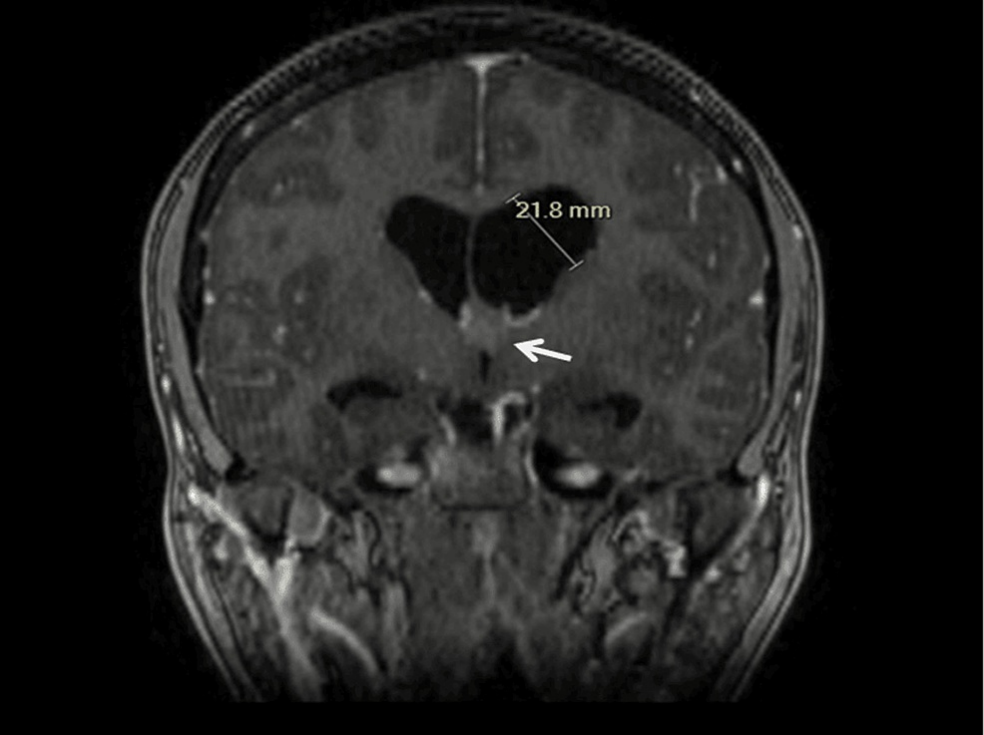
MRI showing an obstructive hydrocephalus measuring 21.8 mm (labelled). A colloid cyst measuring 9 mm is present (arrow).
